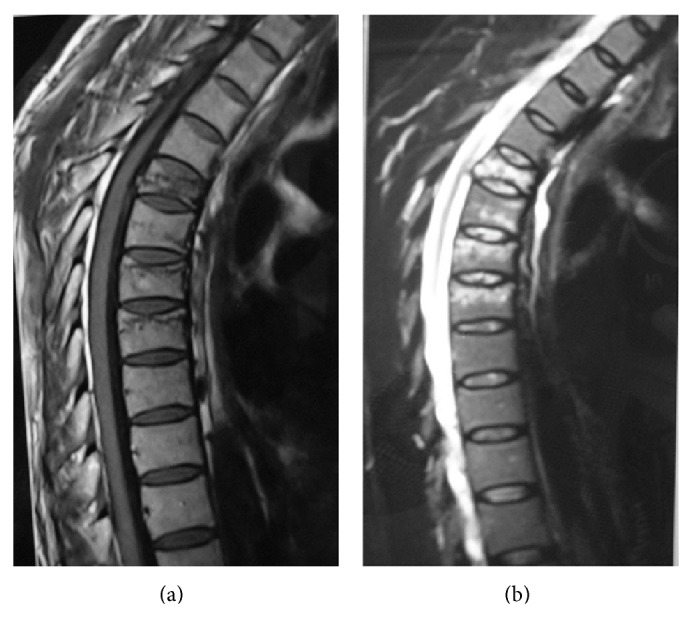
T1-weighted and T2-weighted sagittal magnetic resonance imaging (MRI) of the thoracic spine showing compression fracture at T5, T6, T7, and T8.
